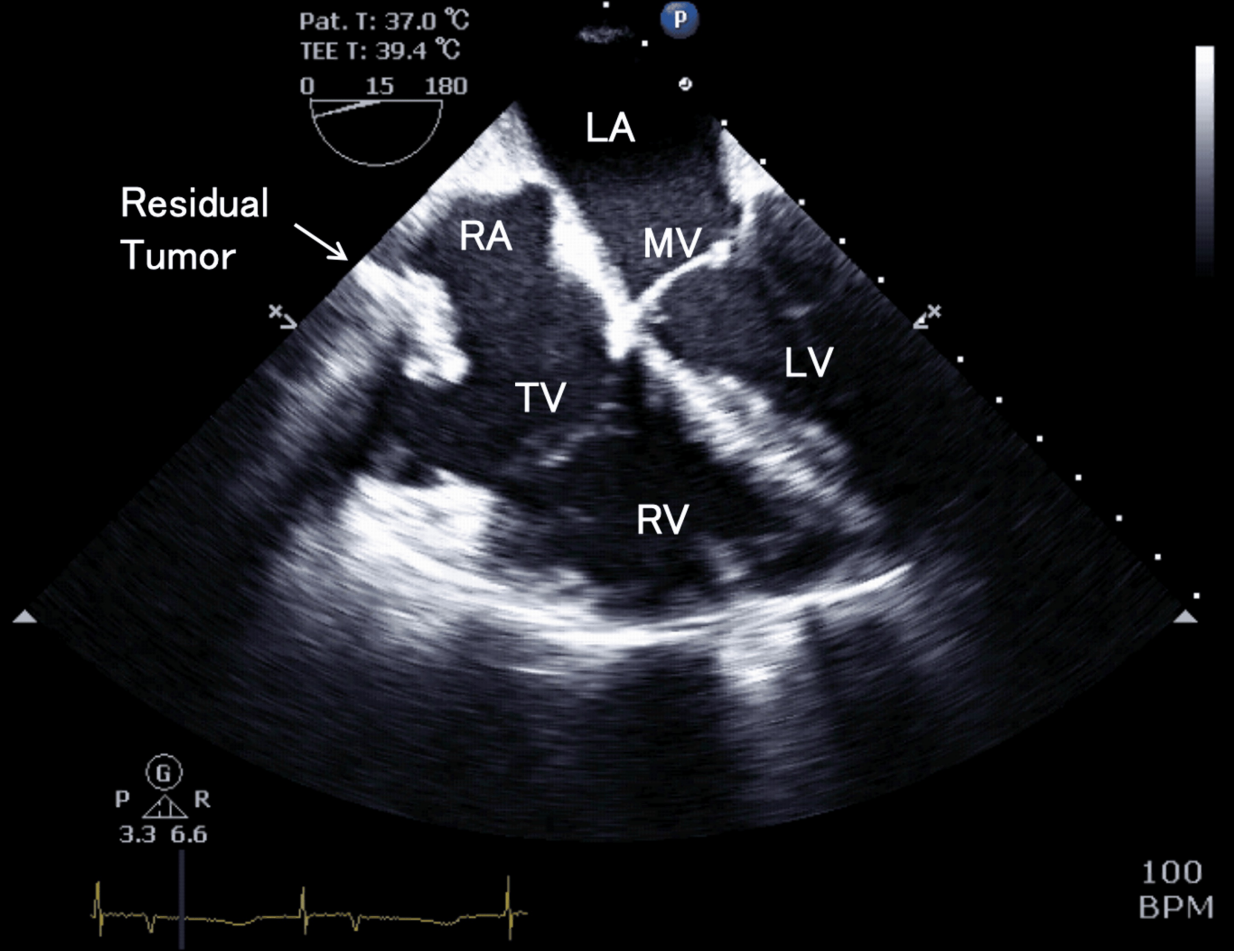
Post-procedural transesophageal echocardiography, four-chamber view This is a four-chamber view image taken after tumor resection and weaning from cardiopulmonary bypass. Although the intracardiac tumor was resected as much as possible, the image shows a residual tumor extending into the inferior vena cava. The right ventricular function was preserved, while the right ventricular cavity remained more prominent than the left. TV, tricuspid valve; RA, right atrium; RV, right ventricle; MV, mitral valve; LA, left atrium; LV, left ventricle
